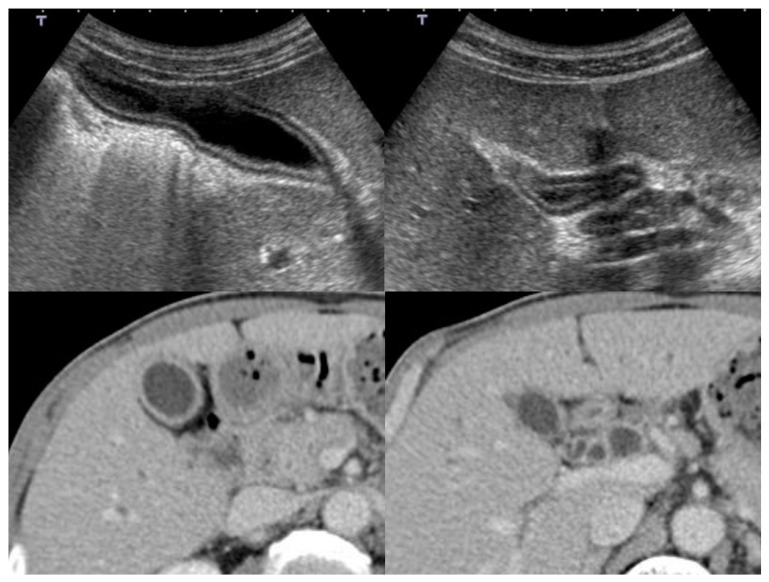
US (3-layer type) and CT (2-layer type) findings of a case with a diffuse type of IgG4-sclerosing cholecystitis.
